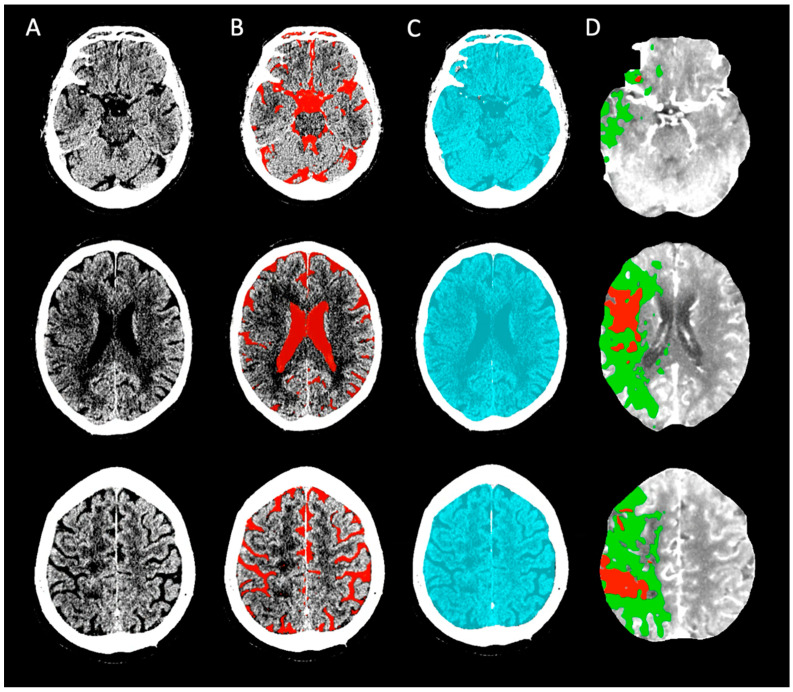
Example of baseline CT imaging with ICV and CSF volume assessments; three levels in the brain are shown. (A) Non-contrast CT of a 71-year-old patient with a right-sided M1 occlusion who received IV alteplase before EVT (eTICI 2b). (B) CSF segmentation (red) shows a CSF volume of 250 mL. (C) ICV segmentation (blue) on baseline NCCT shows an ICV of 1374 mL. (D) CTP-estimated core volume (red) was 50 mL. Penumbral volume (green) was 210 mL. CSF = cerebrospinal fluid; CTP = CT perfusion; eTICI = expanded treatment in cerebral infarction; EVT = endovascular treatment; and ICV = intracranial volume.
